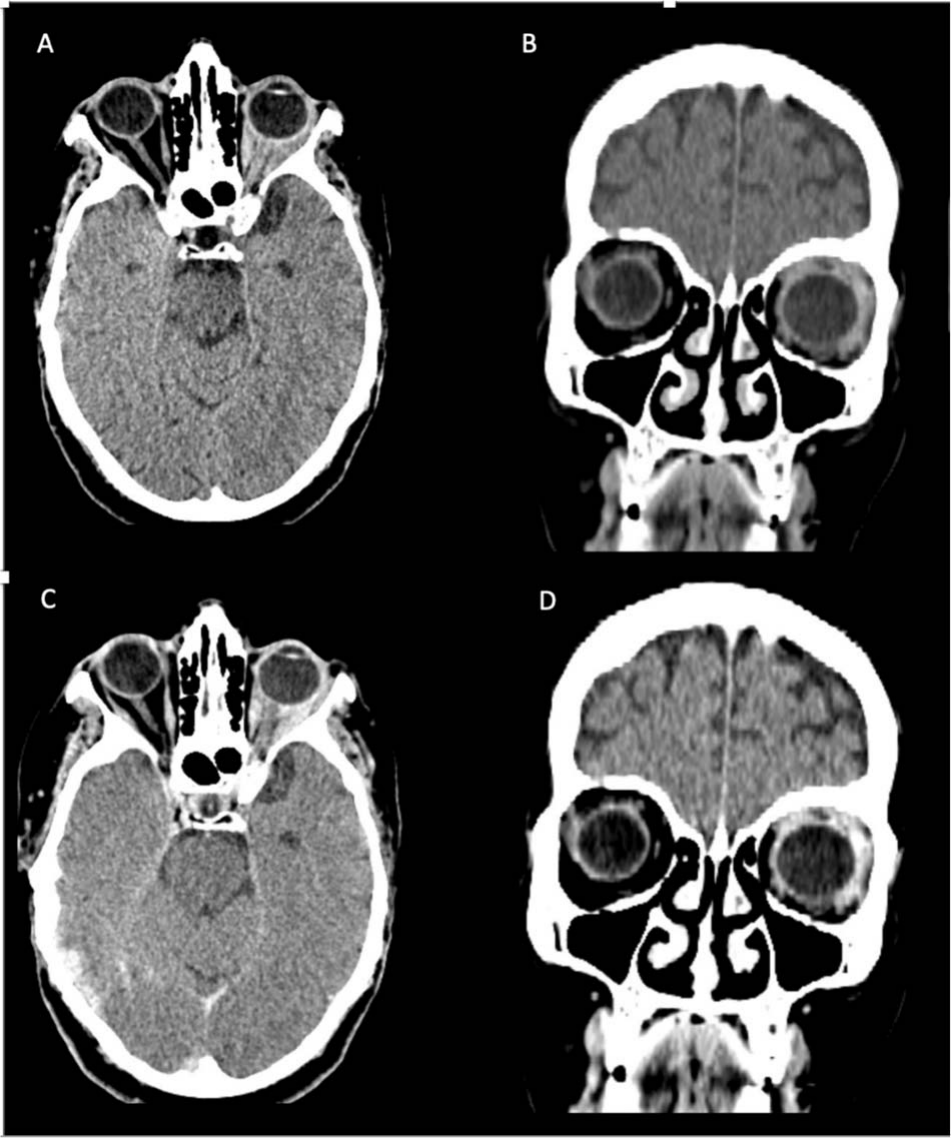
Axial and coronal cerebral CT scans show enlargement of the left lateral and superior rectal muscles (A–B), with slight enhancement (C–D).
